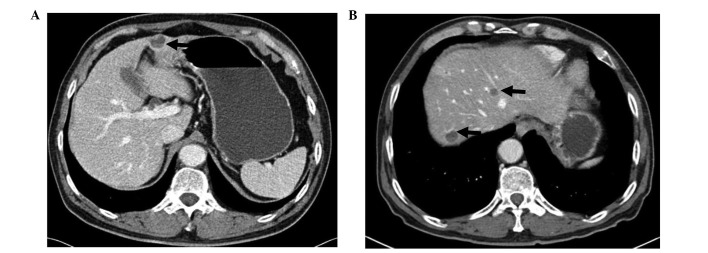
(A) A liver mass ~2 cm in size with heterogeneous internal attenuation was seen by contrast-enhanced abdominal CT (arrow). (B) Follow-up contrast-enhanced abdominal CT showed multiple newly developed liver masses with heterogeneous internal attenuation similar to the initially detected liver mass (arrows). CT, computed tomography.
